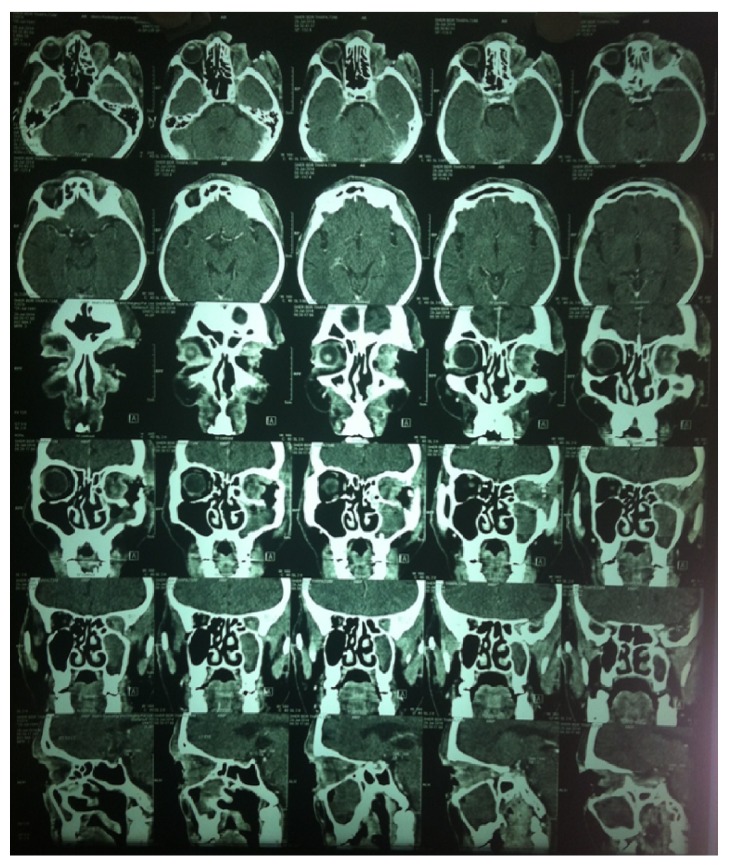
Axial and Coronal section CT scan showing soft tissue density area in left maxillary sinus and left orbit with erosion of the floor and lateral wall of the orbit. No intracranial abnormality seen.
